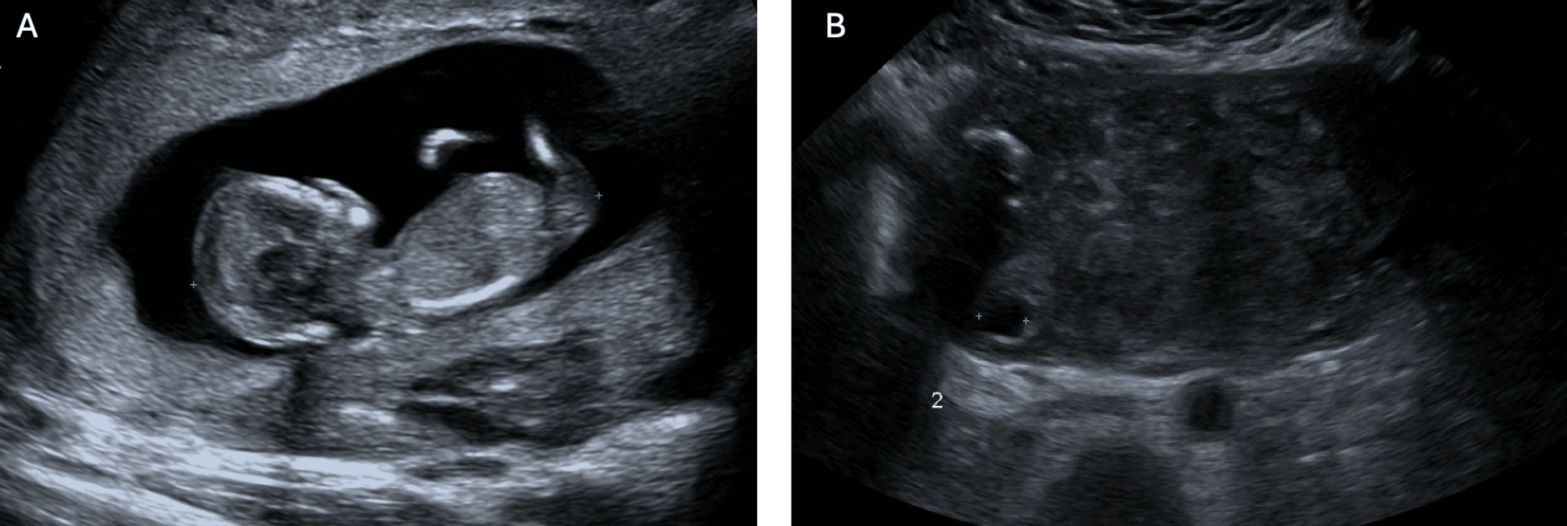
Pelvic ultrasound 28 days post-KCl administration (A) Progressive intrauterine pregnancy that is approximately 13+3 weeks gestation by crown rump length. (B) Previous right cornual ectopic pregnancy with nil further growth (1.4 x 1.1 x 0.9 cm).
